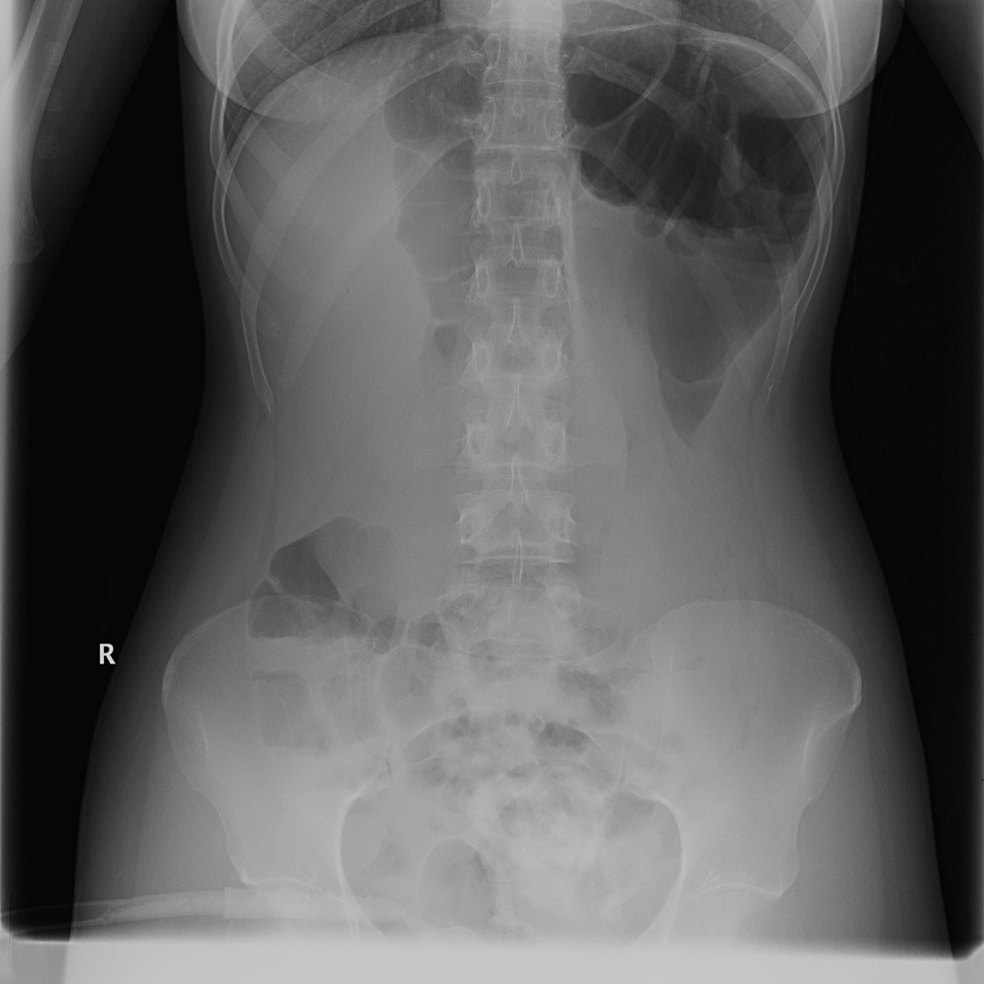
Plain x-ray abdomen suggesting dilated colon without small bowel dilation
